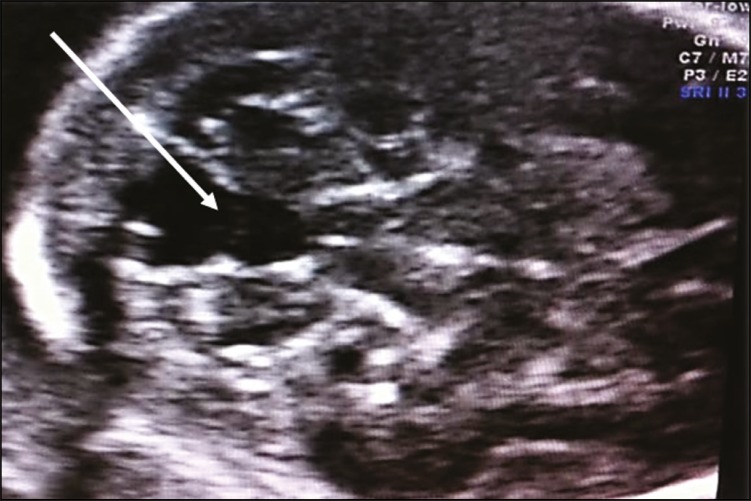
Axial ultrasound of the transcerebellar plane, showing communication between the fourth ventricle and the cisterna magna (arrow).
